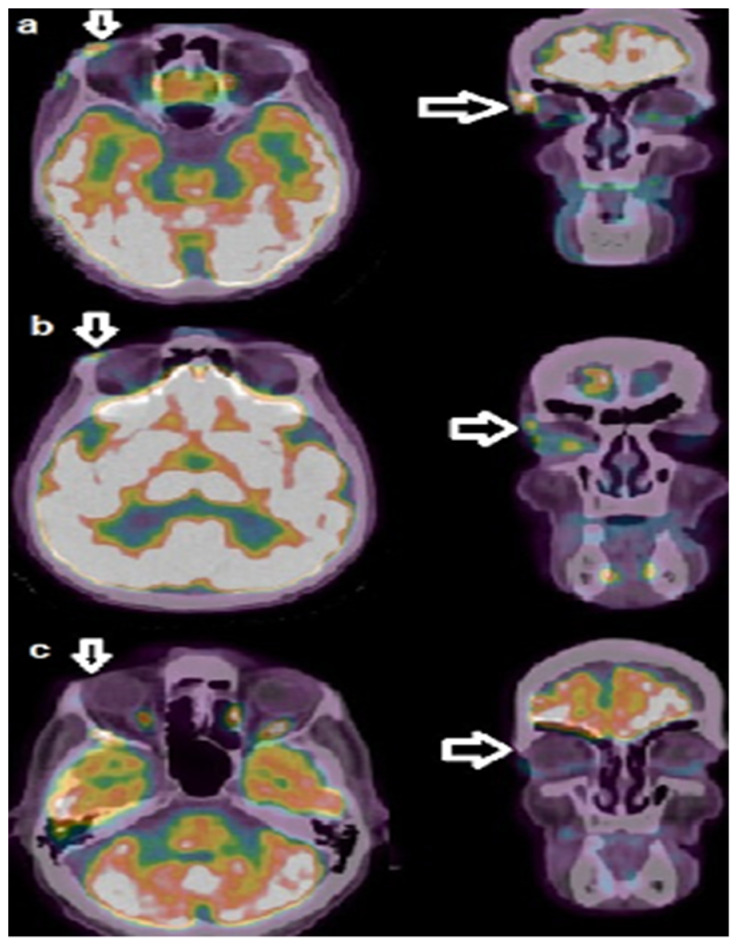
(a) Baseline FDG PET showed a focal area of tracer increased incorporation in the soft tissues adjacent to the supraorbital bone; (b) FDG PET carried at 3 months after Cemiplimab identified a small area of residual tracer uptake in the same region, indicative of a partial metabolic response; (c) FDG PET after 3 years of immunotherapy depicted a complete metabolic response (please refer to arrows).
